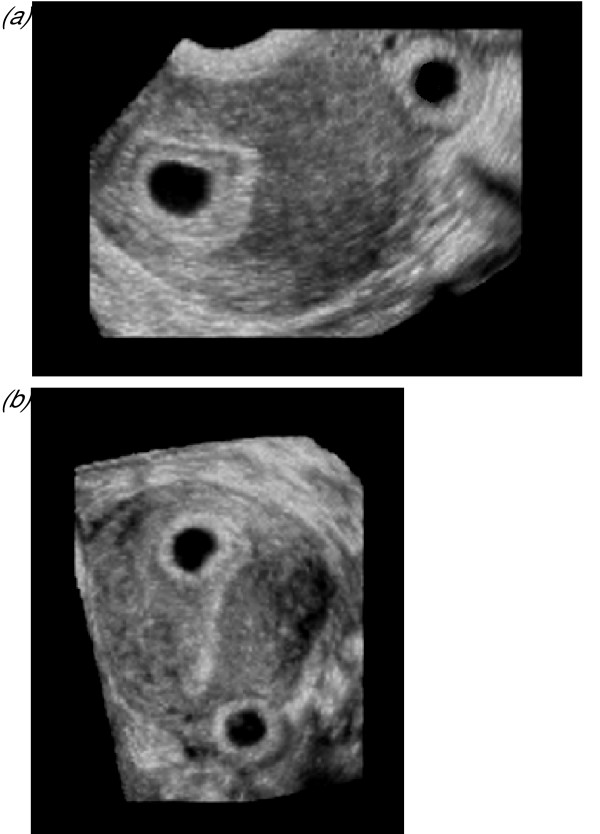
Ectopic pregnancy*. The spatial orientation of three-dimensional ultrasound and its ability to demonstrate the coronal plane of the uterus in its natural position may help in the diagnosis and exclusion of ectopic pregnancy. This is particularly true in cases of heterotropic pregnancy where an intrauterine pregnancy is associated with an ectopic twin gestation. Spontaneous heterotropic pregnancy is rare but may complicate 0.5% of pregnancies arising from IVF treatment with the ectopic gestation located in the cornua (Fig. 13a) or cervix (Fig. 13b).
